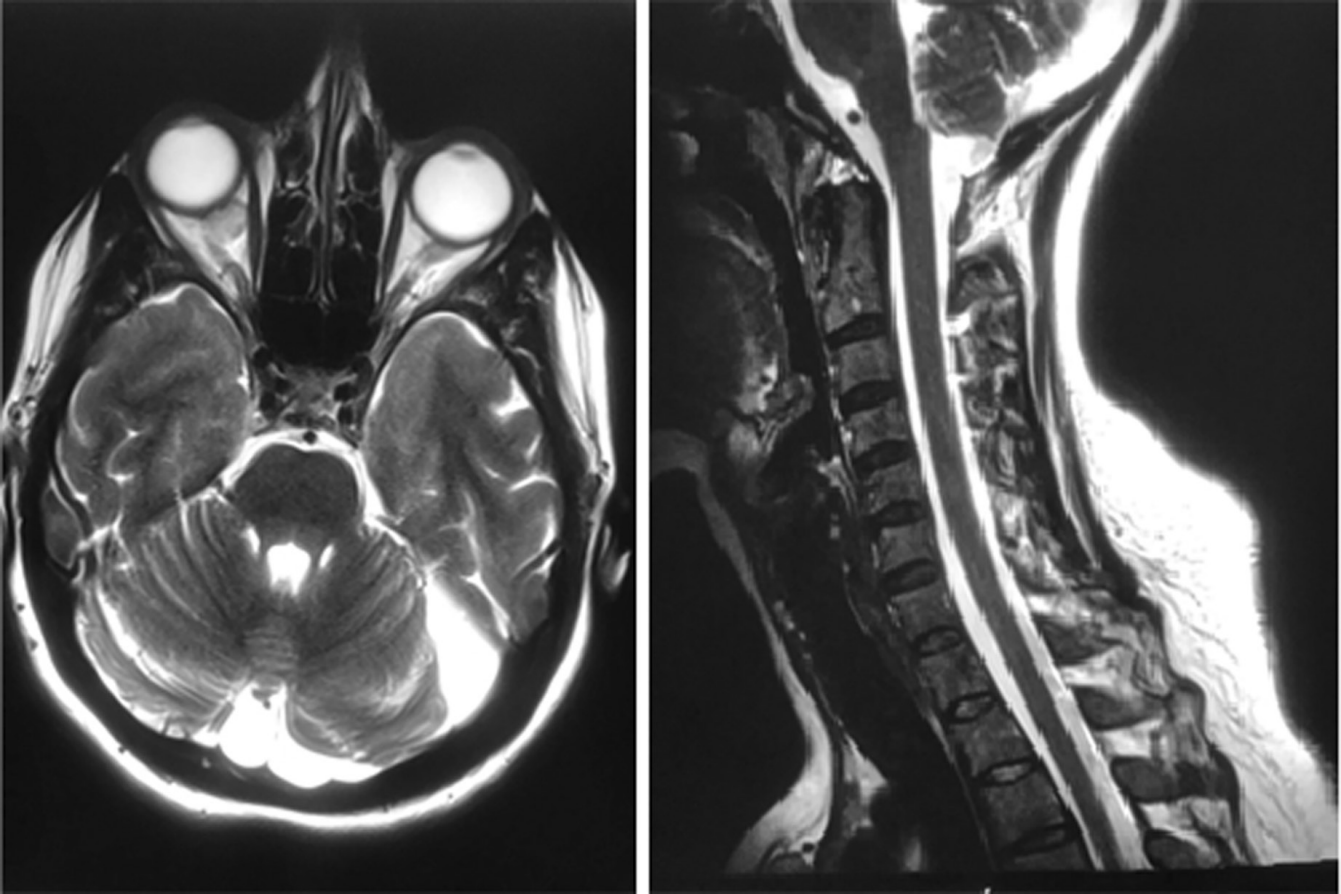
Brain and spine MRI without contrast for the patient.
